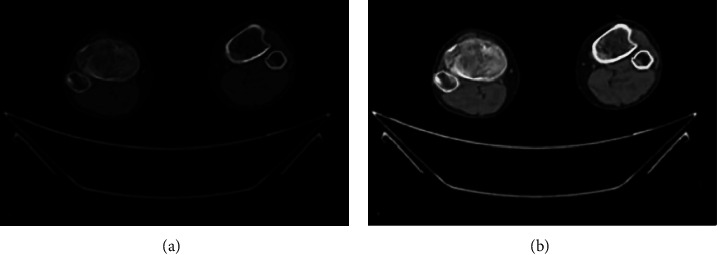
Comparison of CT images after algorithm reconstruction. (a) Original image; (b) reconstructed image.
